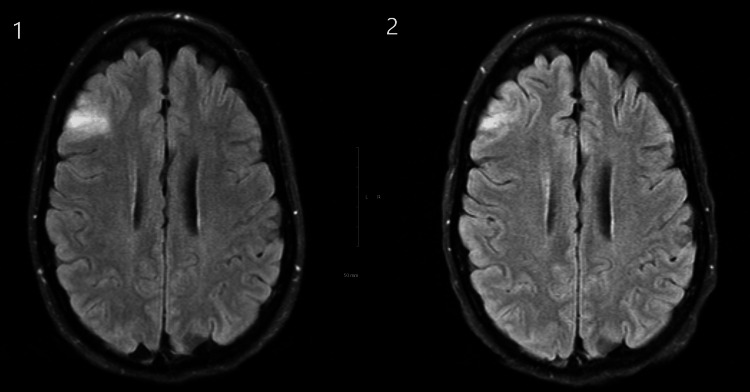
MRI of the brain T2 FLAIR showing hyperintensity in the right lateral frontal lobe during early diagnostic workup (1) and later in the disease course after treatment (2) Treatments included IVIg, plasmapheresis, and rituximab. FLAIR - fluid-attenuated inversion recovery; IVIg - intravenous immunoglobulin
